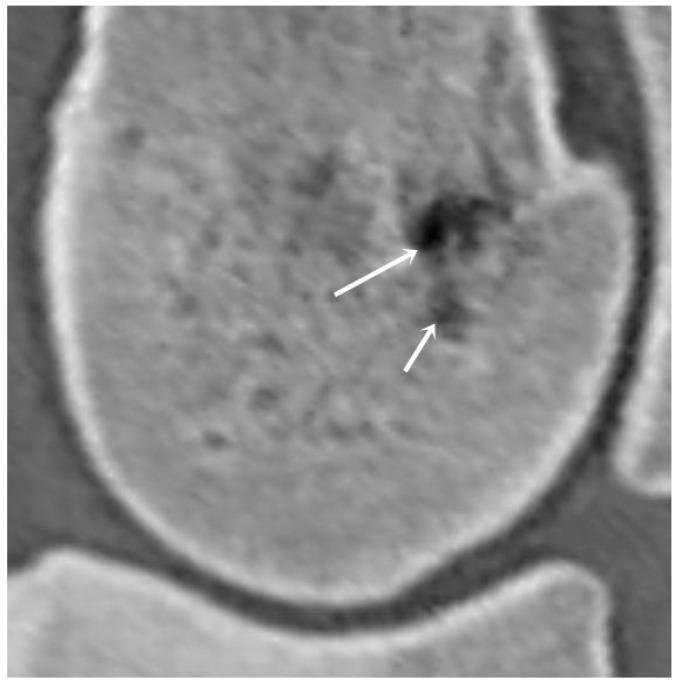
Sagittal plane computed tomographic reconstruction of the lateral parasagittal groove of a third metacarpal bone (dorsal is to the left). The arrows indicate vascular channels, which were documented and classified into three categories based on their size. The diameter of the proximal vascular channel is 3.1 mm, while that of the distal vascular channel is 1.9 mm.
